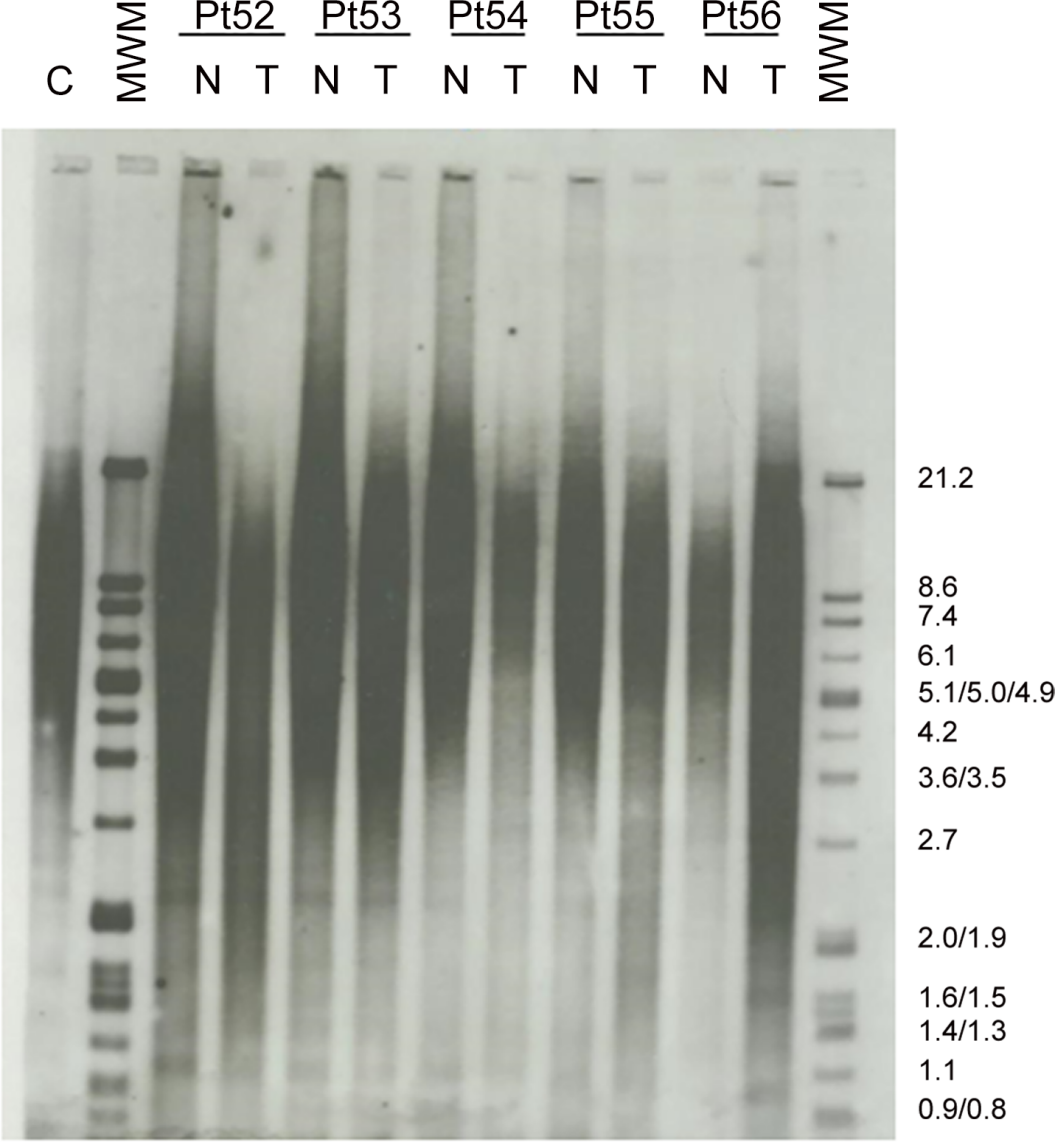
Results of a representative x-ray film for telomere length analysis from five colorectal cancer patients (Pt) in tumour tissues (T) and their paired non-tumour samples (N). C, positive control. MWM, DNA molecular weight marker.
